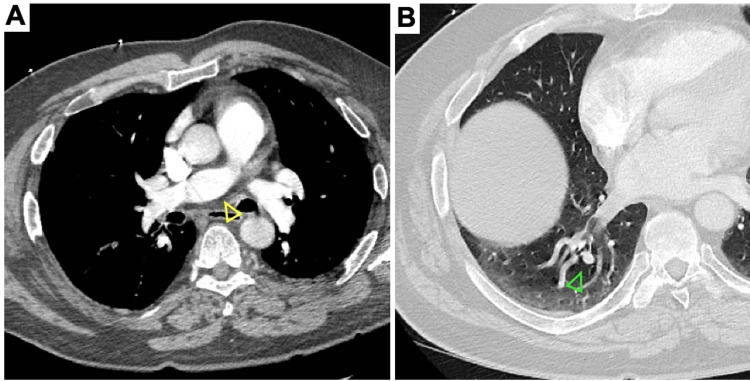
CT angiogram of the chest. (A) Dilatation of the right pulmonary trunk (yellow arrowhead) and (B) intraparenchymal lobar arteriolar ectatic plexus of the right pulmonary vasculature (green arrowhead).
